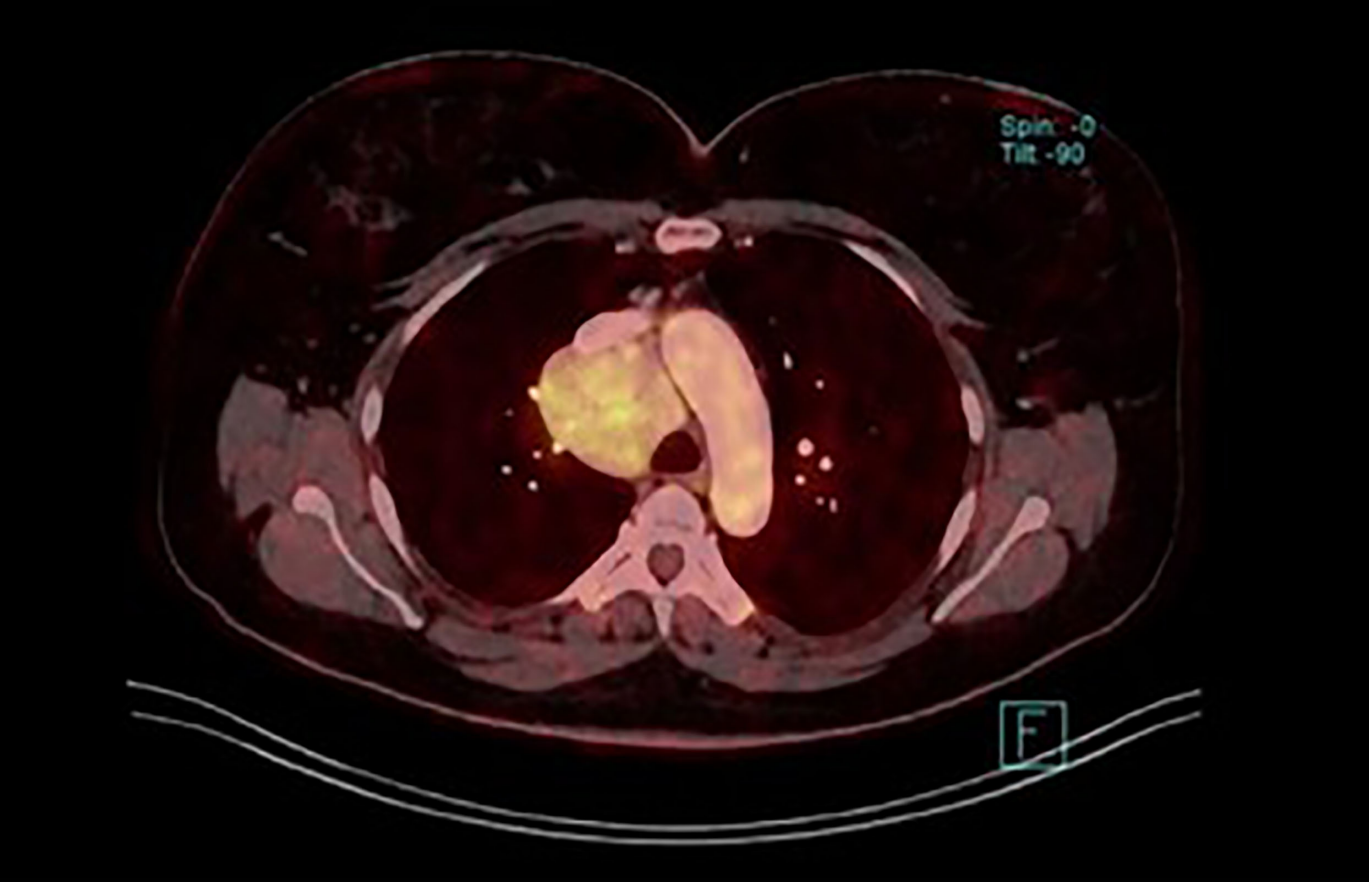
Unicentric CD in the mediastinum (fused image of the fluordeoxyglucose uptake and postcontrast CT from whole body PET/CT scan).
